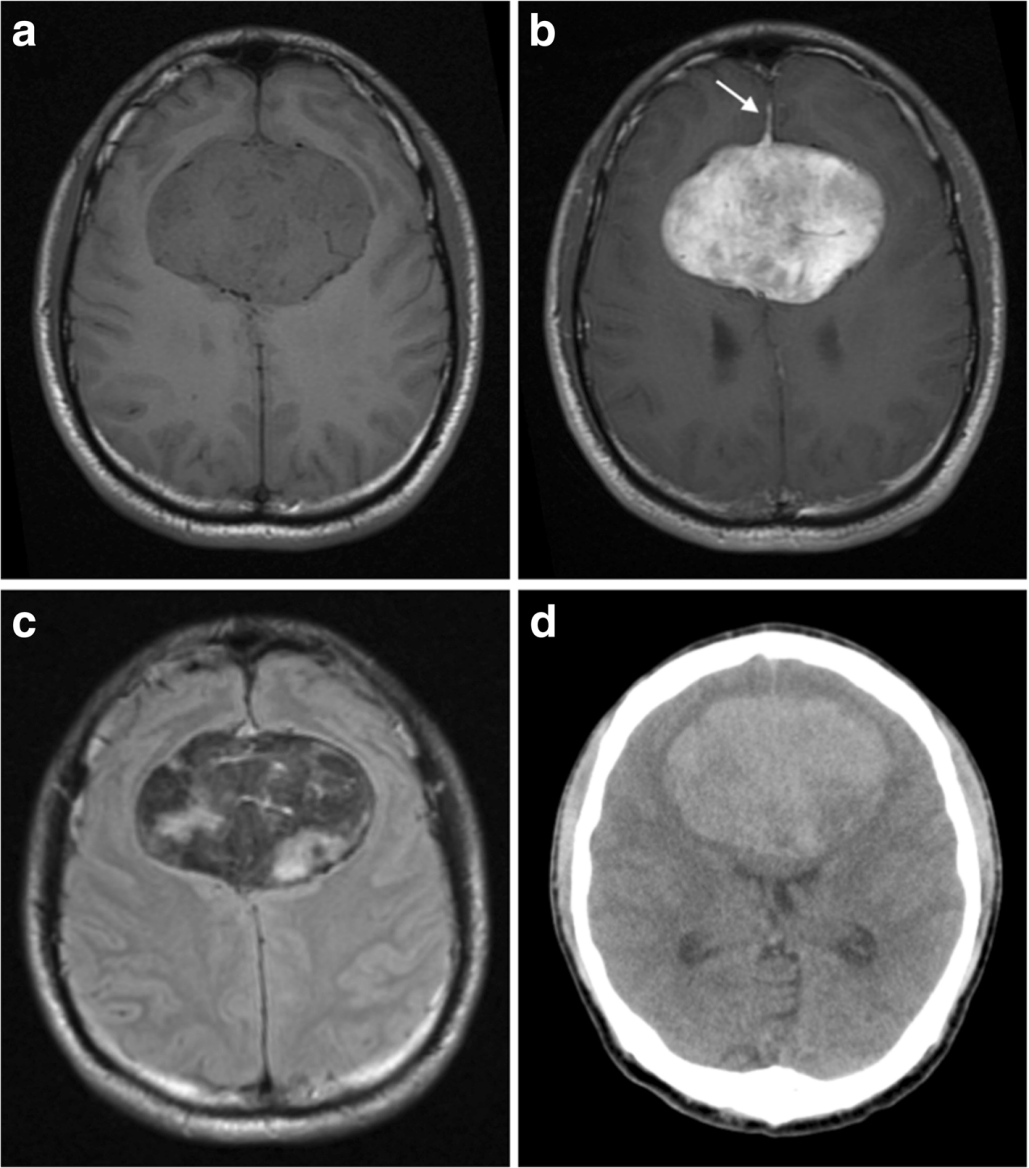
Solitary fibrous tumour. a, b Pre- and post-contrast axial T1-weighted MR images showing an avidly enhancing extra-axial lesion straddling the falx cerebri. An enhancing dural tail is seen anteriorly (white arrow). c On T2 FLAIR images, the lesion has regions of high and low signal consistent with its fibrous collagen composition. Note how these low signal regions enhance with administration of contrast in Fig. 7b. d On unenhanced CT images, the mass is largely hyperdense with no areas of calcification
